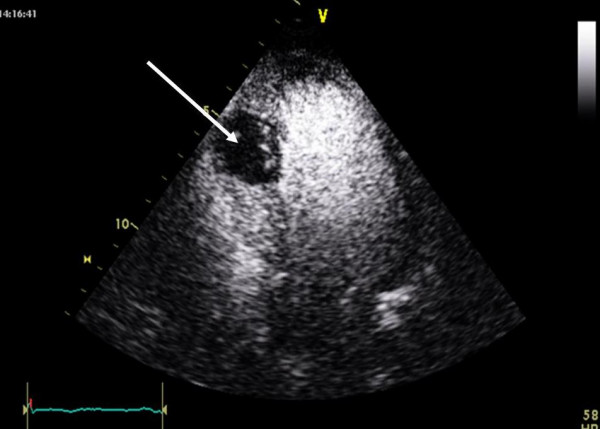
The dark color shows the intraventricular septum thickening (26 mm) and loss of perfusion in the medial septal segment (white arrowhead) demonstrated with low mechanical index contrast echocardiography.
